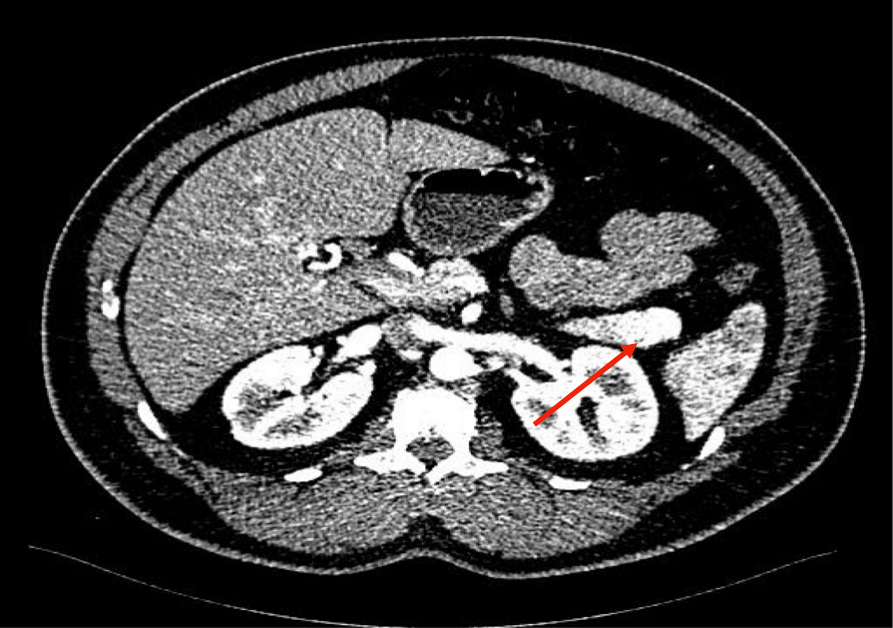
Triple phased abdominal computed tomography scan: arterial phase, axial view. 1.8 × 1.6 hypervascular mass at the tail of pancreas (arrow)
